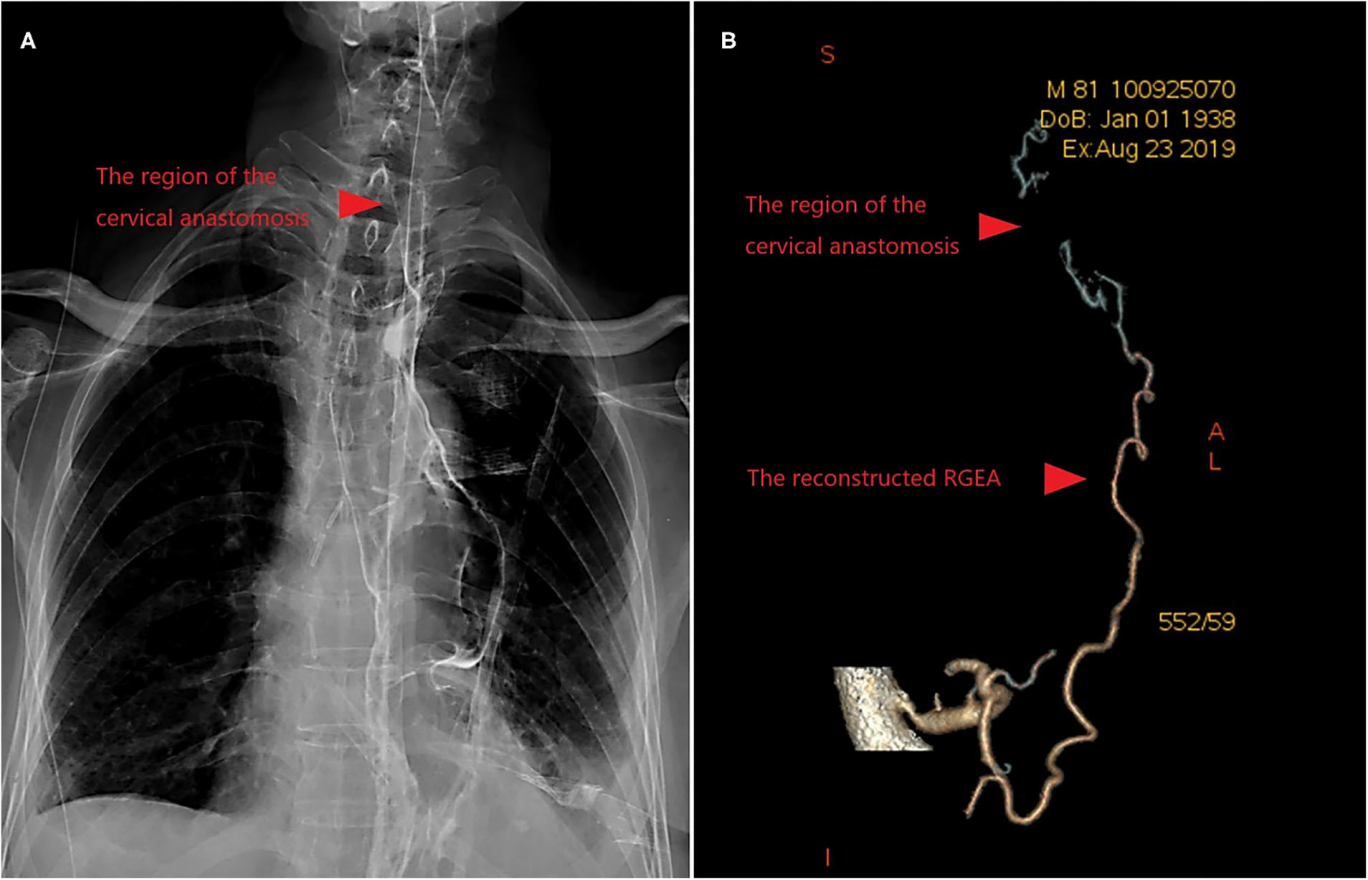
(A) Upper Gastrointestinal Contrast showed that there was no anastomosis leakage after 2 weeks of conservative treatment. (B) The postoperative assessment of blood supply was good revealed by Contrast-Enhanced CT.
